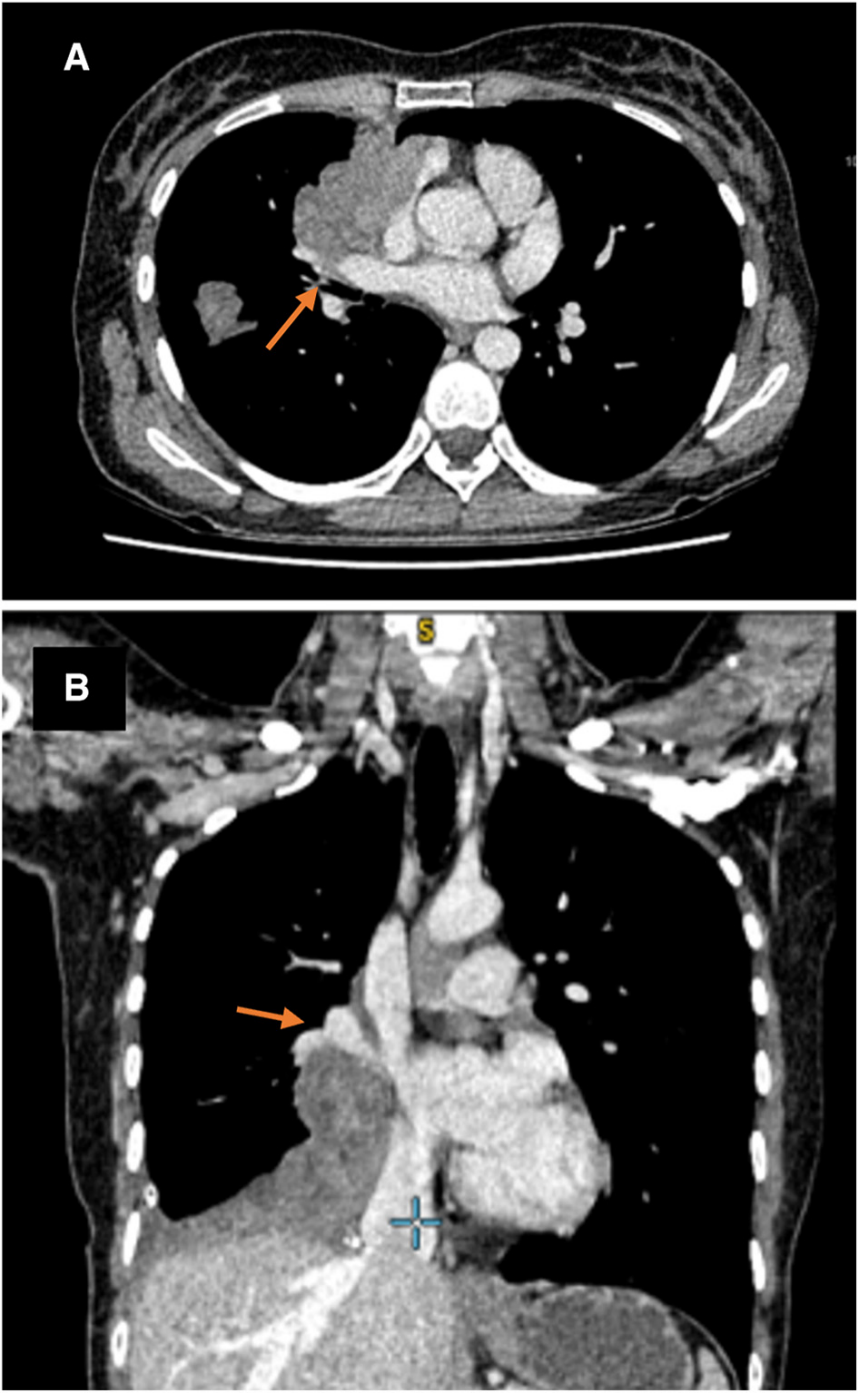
Axial computed tomography scan of the chest with contrast. Arrows show involvement of right main pulmonary artery at origin of upper lobe (A); and effacement of inferior pulmonary vein found to be involved intraoperatively (B).
